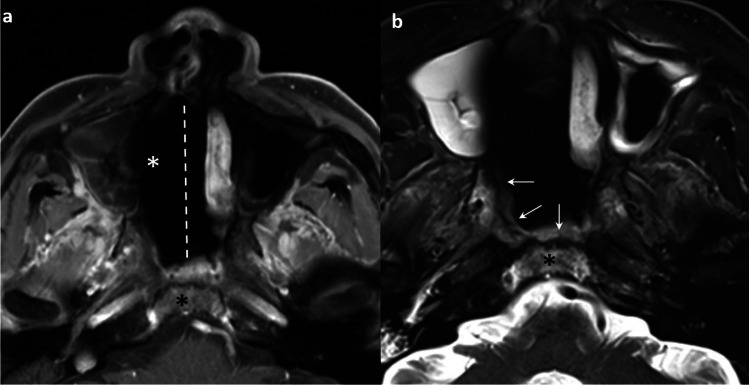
Expected post-operative changes in a 51-year-old man who had NPC (clinical T3N2M0) and completed chemoradiation in 2022. He had recurrent NPC (rT1N0M0) one-year after completion of treatment and underwent transnasal endoscopic nasopharyngectomy. A surveillance MRI was performed at 6-month post-surgery. Axial T1-weighted image with fat suppression shows the posterior septectomy of the nasal septum (dashed line) and the absence of right inferior turbinate in keeping with inferior turbinectomy (white asterisk). The clivus shows diffusely increased T2-weighted signal suggestive of marrow edema (black asterisk) (a). Axial T2-weighted image with fat suppression shows the nasoseptal flap covering the bony defect of the bilateral posterior wall and right lateral wall of the nasopharynx with a deeper hypointense layer and a more superficial smooth thin T2-weighted hyperintense layer (arrows). The right torus tubarius has been resected. There is also enhancing marrow edema in the clivus related to bone drilling, in keeping with post-operative change (asterisk) (b)
